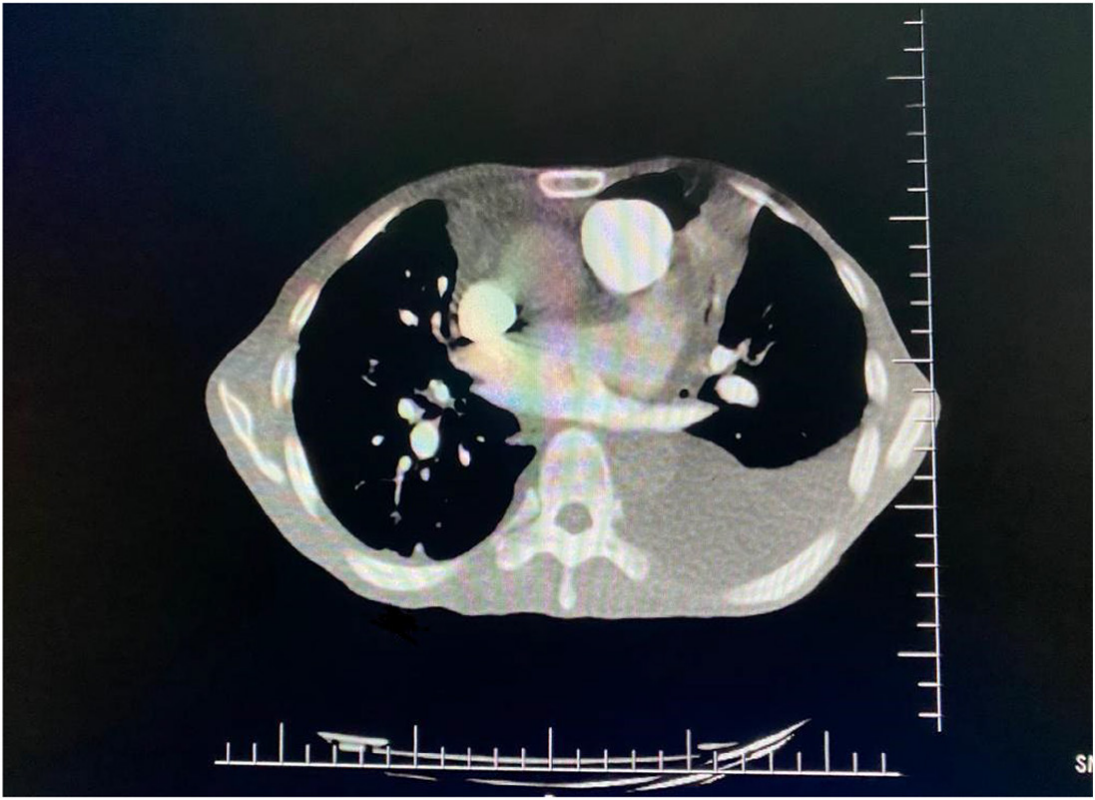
Computed tomography pulmonary angiogram (CTPA).
